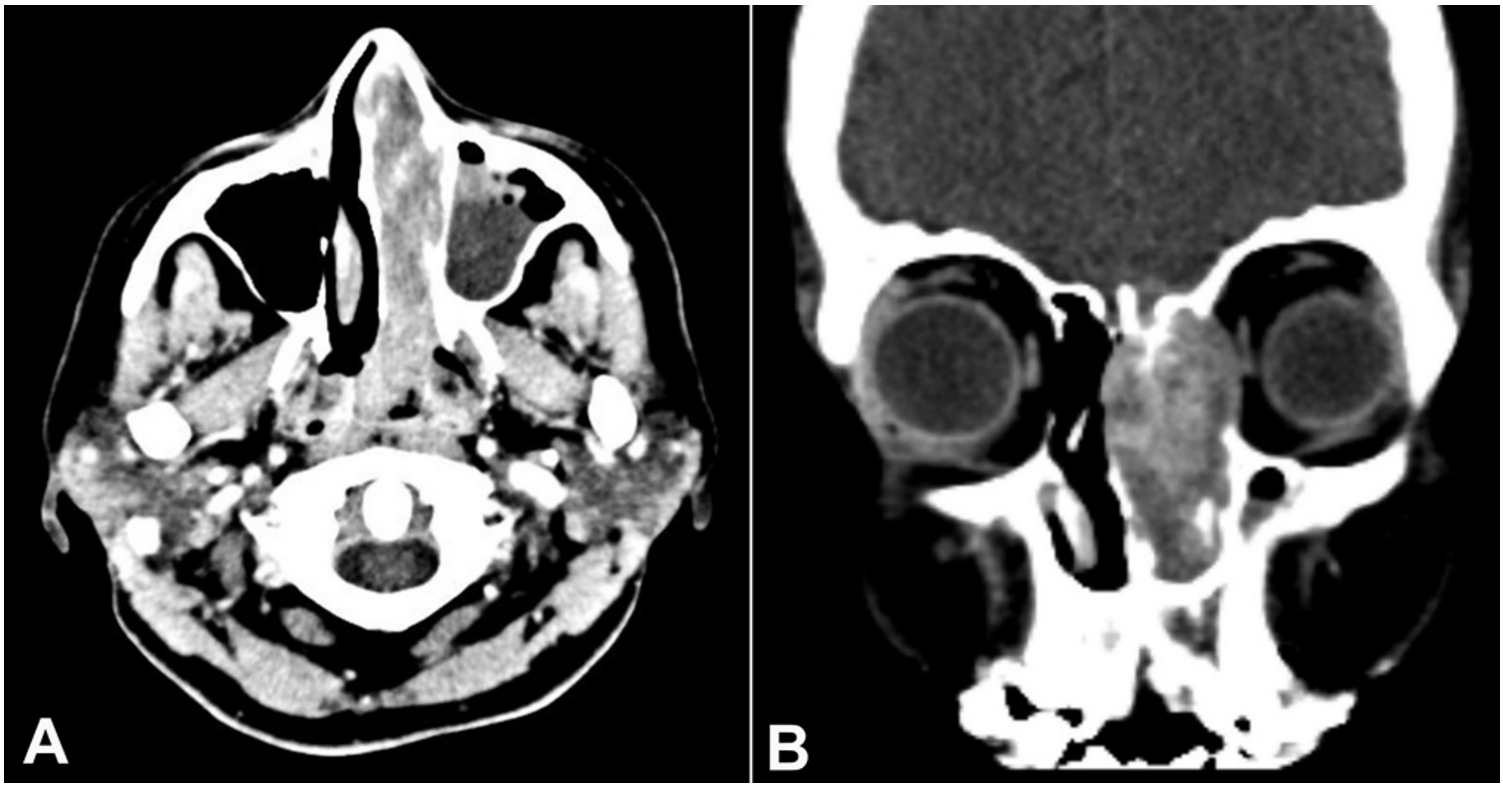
Non-enhanced CT scan showed in: A - an expansile soft tissue mass occupying the left nasal cavity, with hyperdensity and extending to the nasopharynx with a significant invasion to the left maxillary sinus; B - the mass showed close contact with the medial rectal muscle of the left eye.
